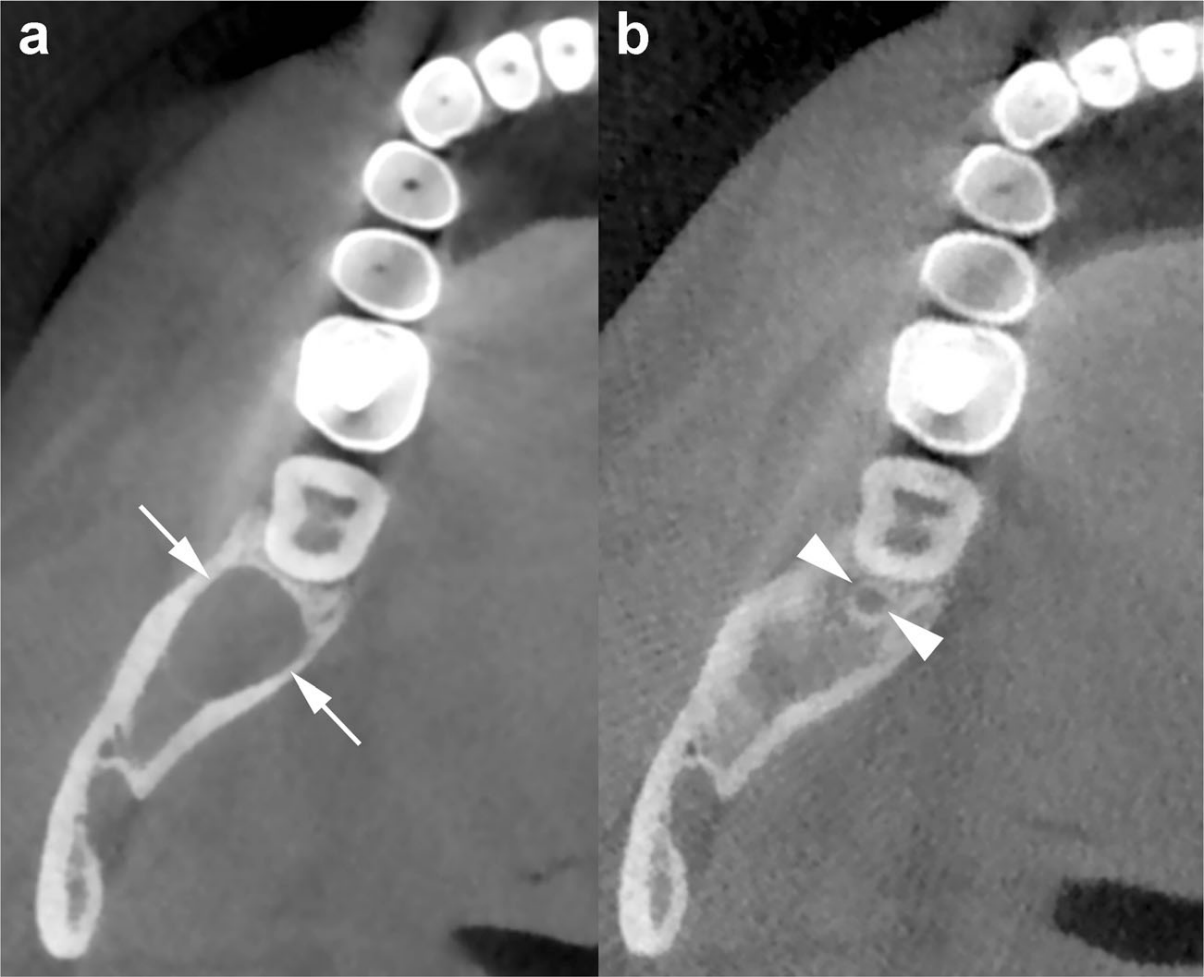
Periodic follow-up of the same patient in Fig. 16 with NBCCS. a Axial CBCT image shows a new lesion in the third molar region of the right mandible (*arrows*), which was subsequently surgically treated. b A postoperative axial CBCT image obtained 1 year later demonstrates a very small recurrence at the site of the previous lesion (*arrowheads*)
